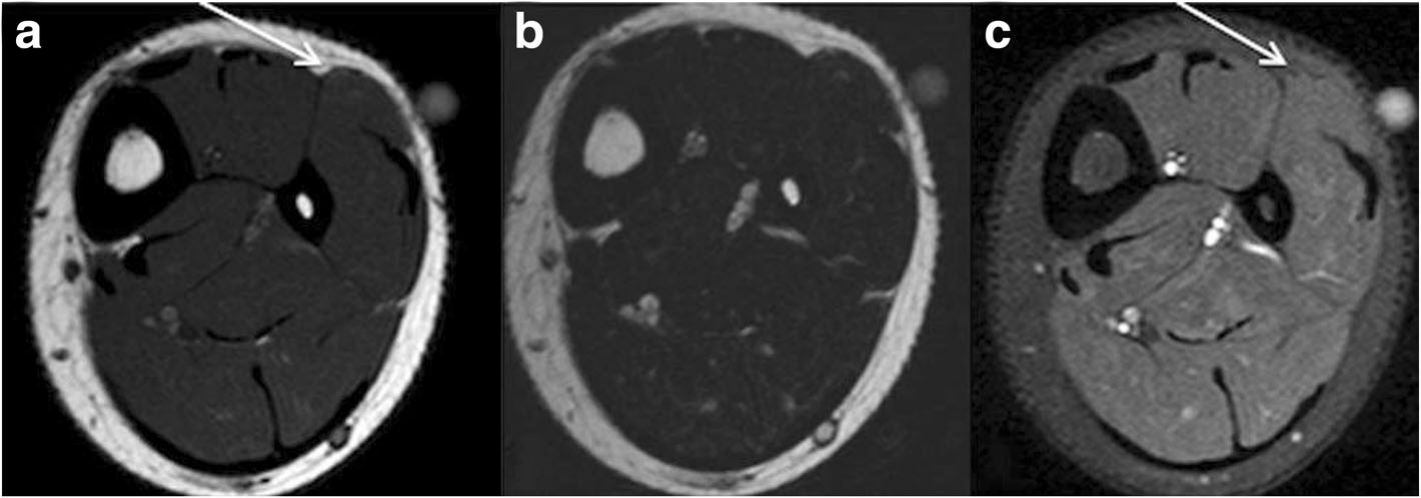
A 16-year-old kickboxer with a suspected “tumor” of the lateral leg, location marked with a capsule. Axial T1 (a), T2 (b), and post contrast T1f-weighted (c) MR images demonstrate a muscle hernia of the peroneus longus muscle with outpouching of the muscle through the antero-lateral investing fascia. The hernia follows the imaging characteristics of the muscle tissue in all sequences. There was a history of direct trauma while playing soccer 1 year ago, presumably resulting in a tear of the investing fascia. The patient noted an increase in size during training/muscle activity. *Reprinted by permission from Springer: Springer Nature, Magnetic resonance imaging of the skeletal musculature, MRI of muscle injuries, Simon Dimmick, Christoph Rehnitz, Marc-André Weber, and James M. Linklater, 2014*
